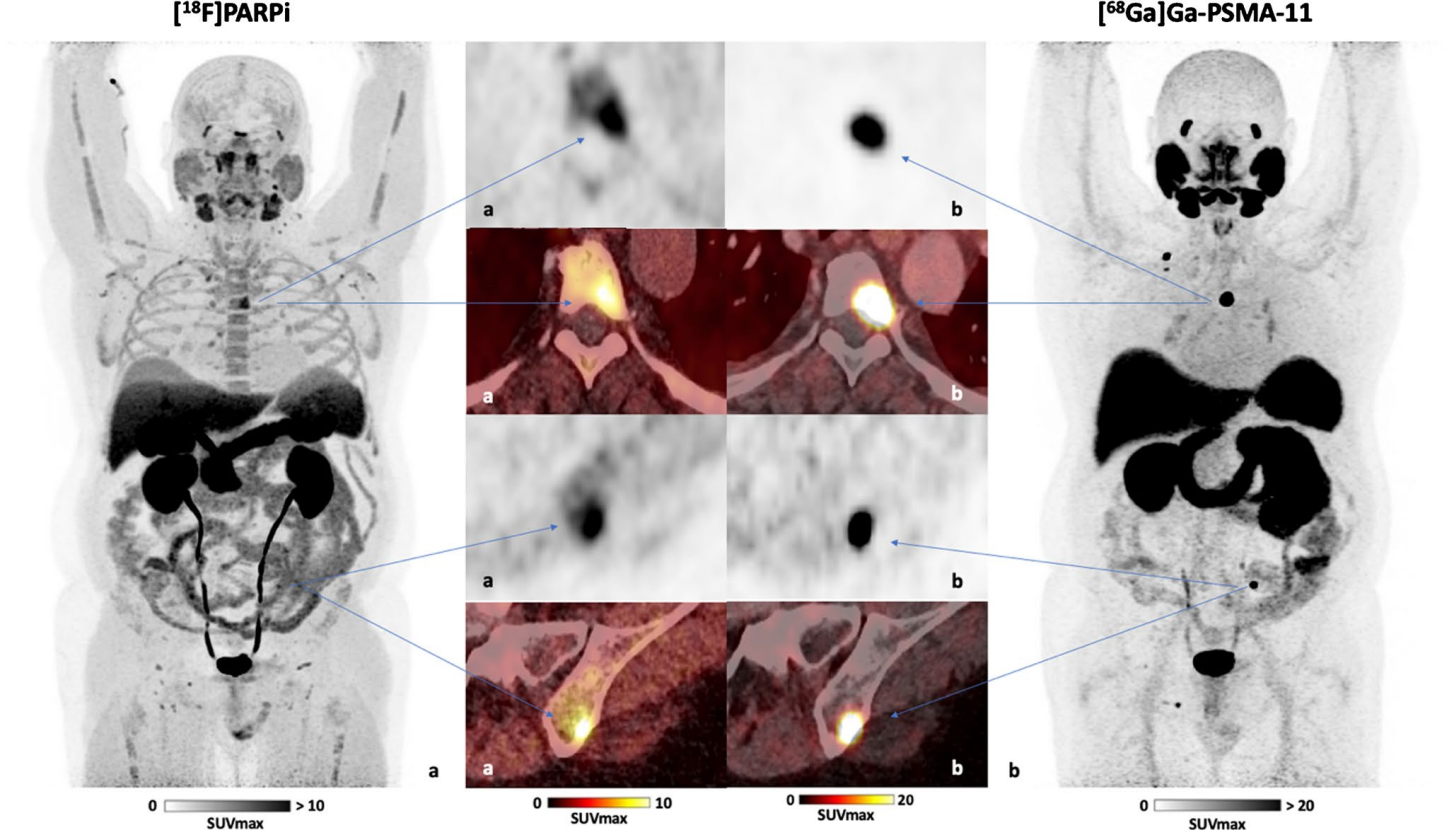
Maximum intensity projection (MIP) PET images of patient B: PET/CT images of a 55-year-old patient with metastatic castration-resistant prostate cancer (mCRPC) who has a confirmed BRCA2 mutation (HRD-positive) and a PSA level of 0.2 ng/ml at the time of imaging. Some bone metastases were identified using (a) [^18^F]PARPi PET/CT, showing a maximum standardized uptake value (SUV_max_) of 6.5, and (b) [^68^Ga]Ga-PSMA-11 PET/CT, with an SUV_max_ >50.0
